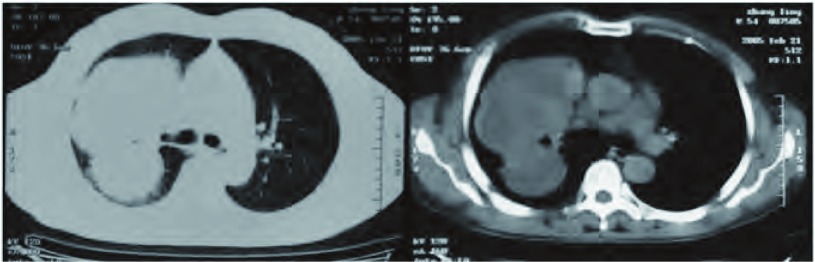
CT检查（2005年2月）：右上叶肺周边部软组织影9.5 cm×12.8 cm，分叶状、部分短毛刺，外侧缘贴近壁层胸膜，内侧缘与肺门结构分界不清，平均CT值24 Hu。 CT scan of the chest (February, 2005): A tumor size is 9.5 cm×12.8 cm at the superior lobe, its lateral edge closes the pleura and the adhesion to the chest wall is seen, the inside edge closes to the hilum of right lung and the boundary is not clear, the lobulated and blurry verge of the neoplasm could be observed with short burr, CT value is of 24 Hu.
